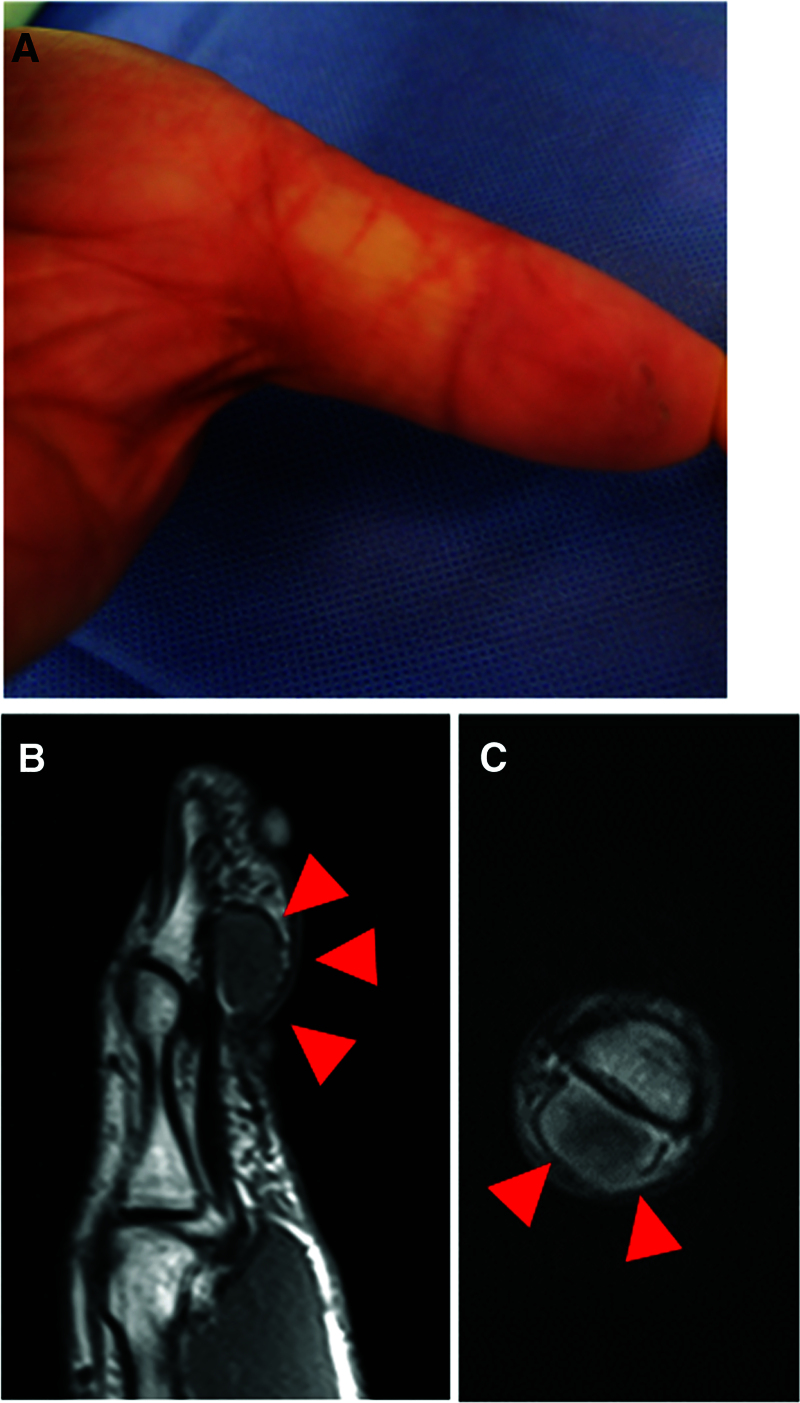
(A) Appearance of the left thumb at the first visit. There is no obvious swelling or abnormal pigmentation. (B) Sagittal T_1_-weighted MRI of the left thumb. A low-intensity mass is observed on the subcutaneous palmar side of the distal thumb (red arrowheads). (C) Coronal T2-weighted MRI of the left thumb. The iso-low-intensity mass is on the subcutaneous palmar side of the distal thumb (red arrowheads). MRI = magnetic resonance imaging.
